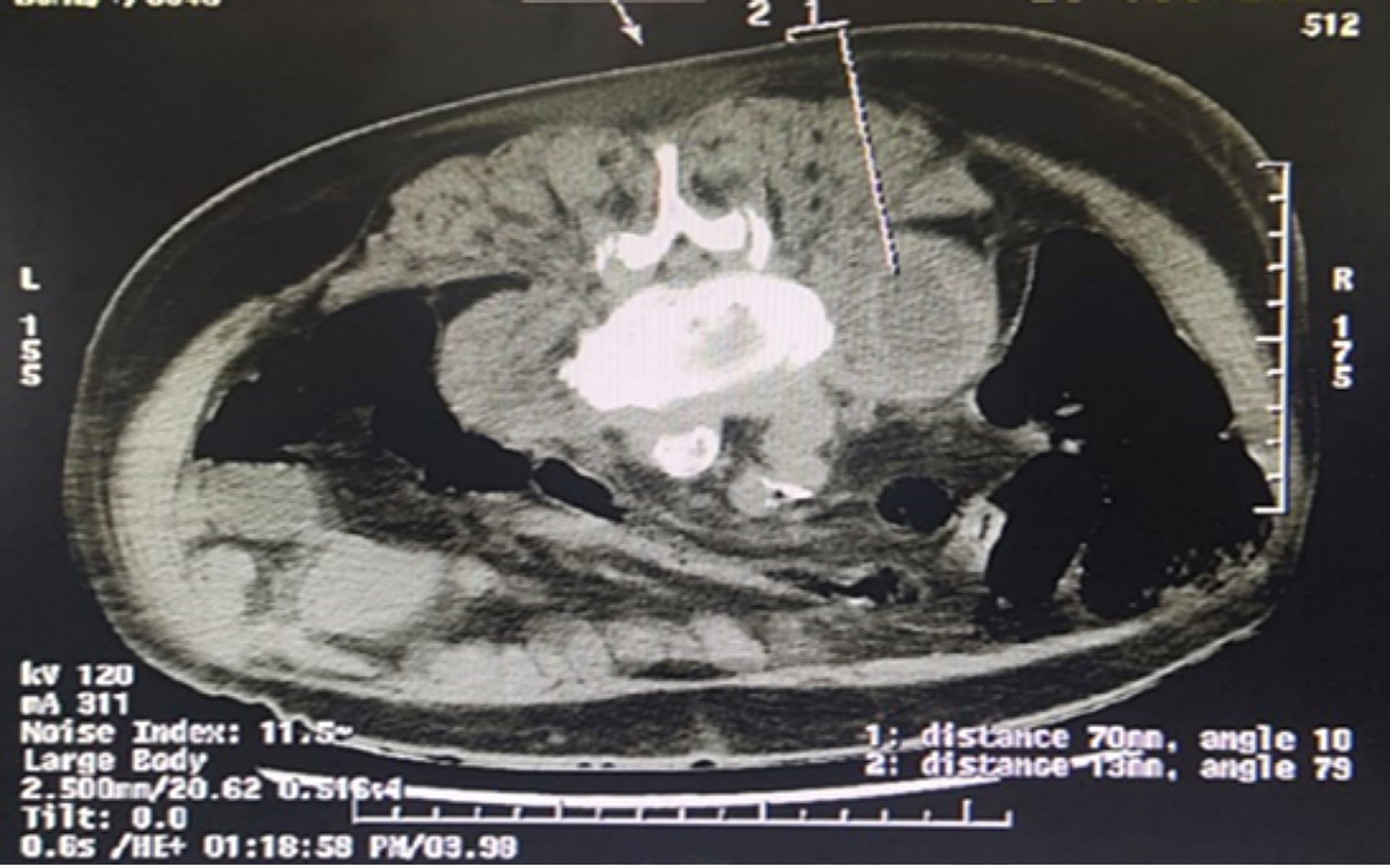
CT-guided aspiration/biopsy of the right-sided psoas abscess.
